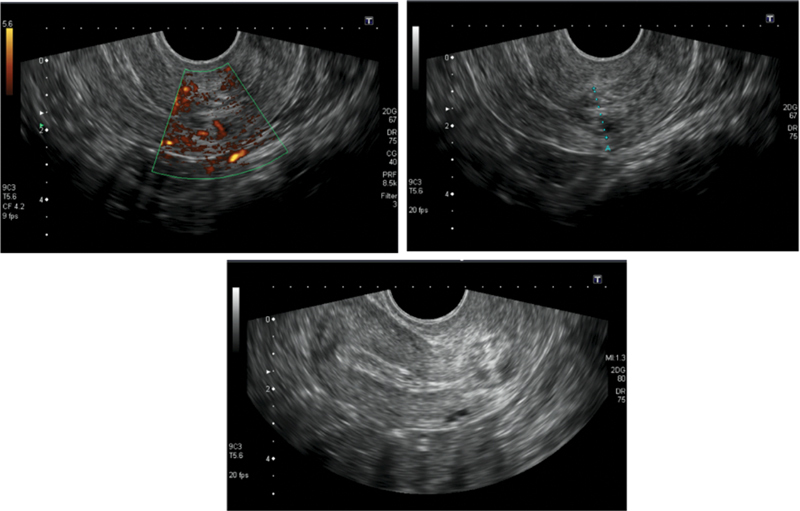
Ultrasonography aspects of cervical ectopic pregnancy.
